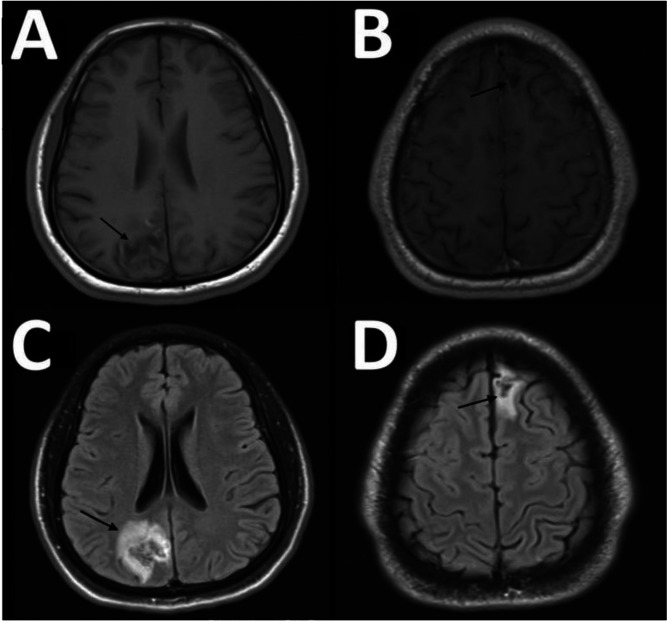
MRI head T1 (A, B), Flair (C, D) showed two lesions (black arrows) with heterogenous area seen mainly in subcortical and abutting cortical noted on right occipital parietal and anterior paraflacine left frontal lobe with surrounding marked vasogenic edema
